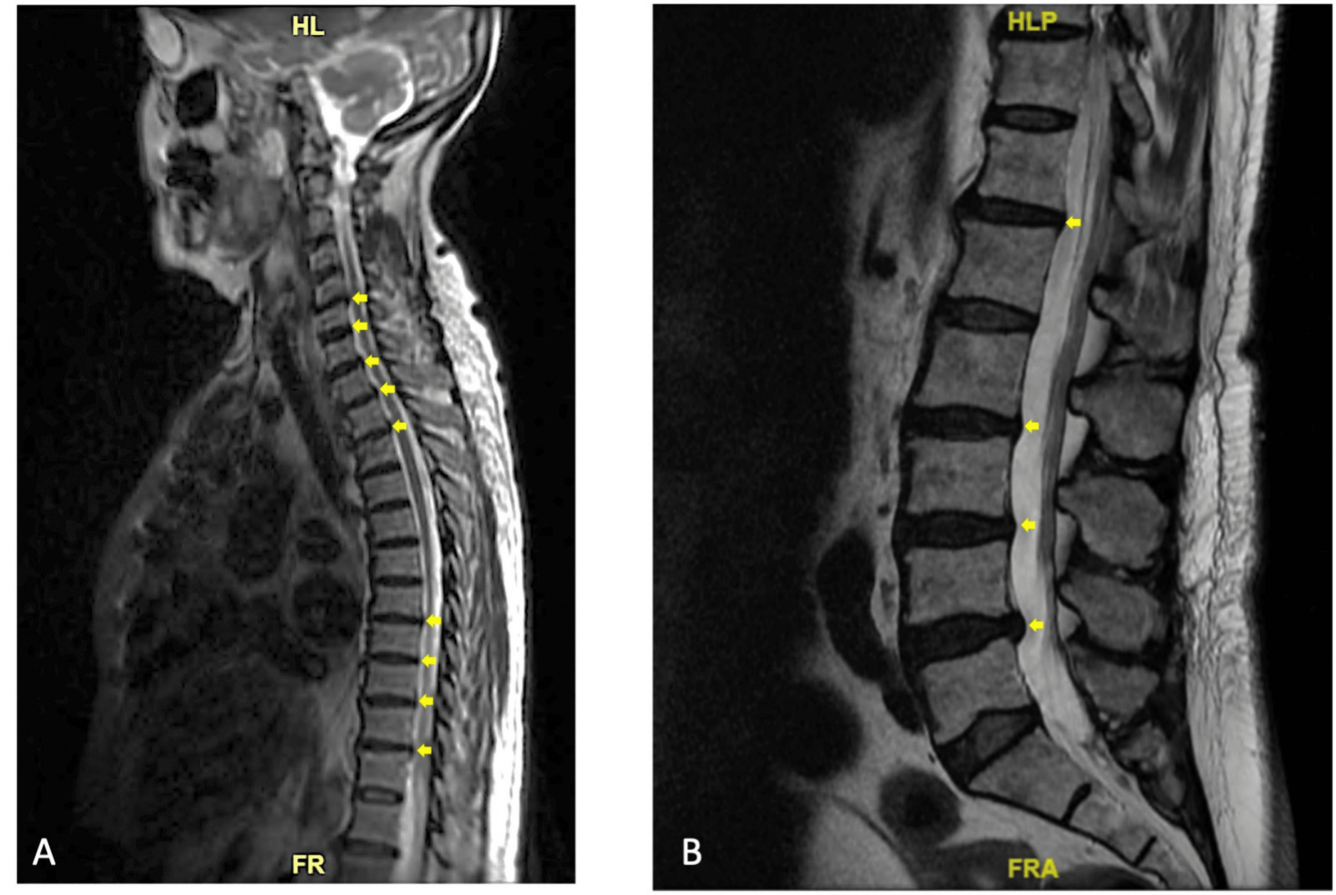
MRI of the thoracic and lumbar spine demonstrated degenerative changes with no evidence of severe spinal canal stenosis. A: MRI thoracic spine; B: MRI lumbar spine; Yellow arrows: small disc bulges.
